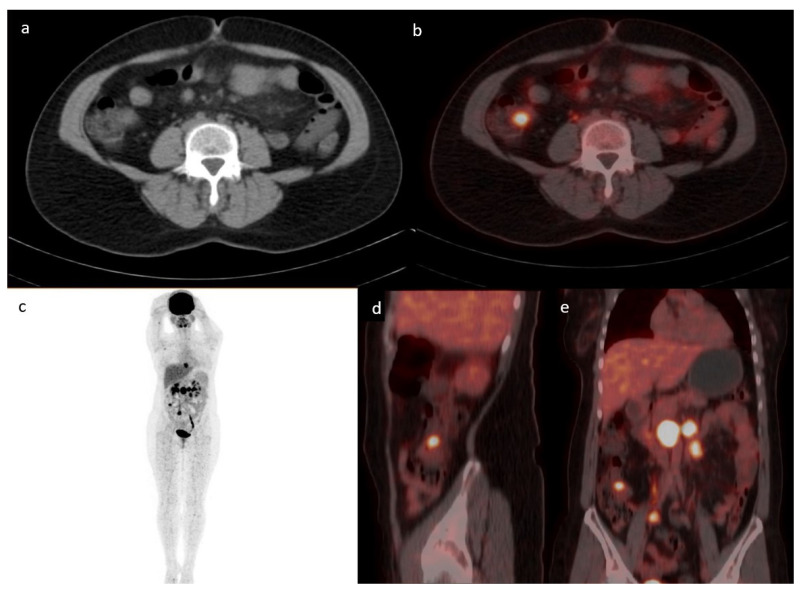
Case of a 43-year-old female with a history of follicular lymphoma. Axial CT (a) fused with PET (b) showing a focus of increased uptake at the level of the ileocecal valve with an SUVmax of 7.1 corresponding to a 1.7 cm soft tissue density. MIP (c) showing multiple active supra and infra diaphragmatic lymph nodes. The infra diaphragmatic lymph nodes are seen again in the fused sagittal (d) and coronal (e)-fused PET/CT images. The patient underwent a colonoscopy where ileal biopsy showed non-necrotizing granulomas, with no evidence of lymphoma or microorganisms.
